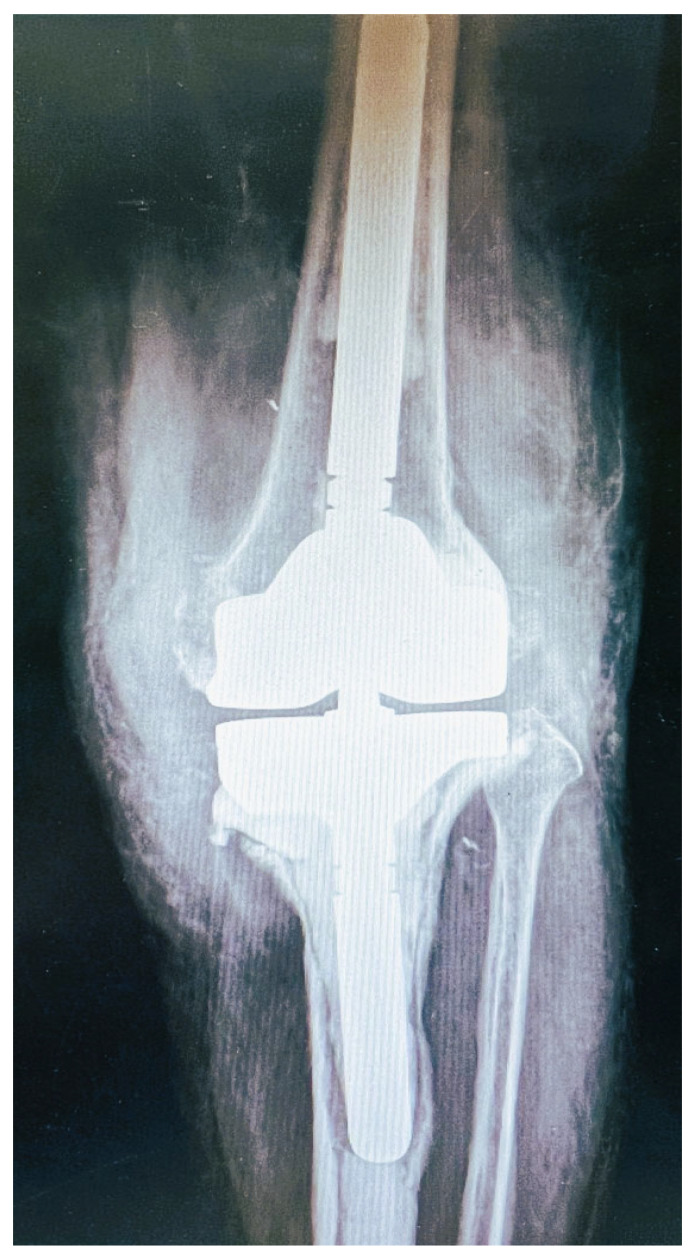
Pre-operative X-ray: anteroposterior view of the revised knee arthroplasty. Loosening is evident especially at the tibia component.
